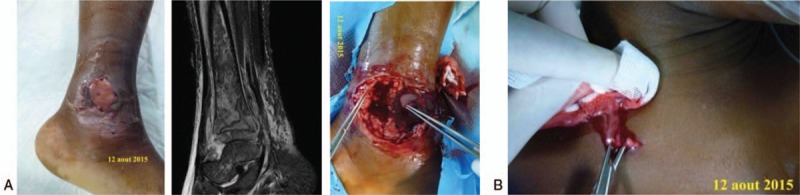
(A) Acute hematogenous osteomyelitis and ankle septic arthritis, before and during surgery. MRI, sagittal plane, STIR. (B) Pyomyositis.
